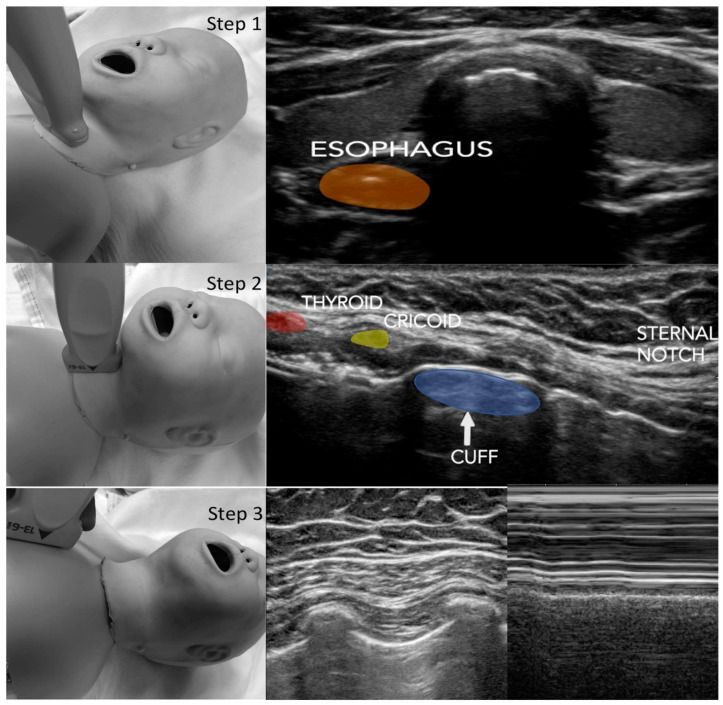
PLUS (pulmonary tree and lung expansion ultrasound study). Step 1: Trachea/Esophagus Assessment—The ultrasound probe is placed transversely on the anterior neck approximately 2 cm superior to the suprasternal notch and scanned cranially/caudad. Step 2: The probe is then rotated 90 degrees such that the long axis of the trachea is achieved with the cricoid cartilage. Step 3: Pleural Sliding Assessment—The ultrasound probe is placed vertically on the anterior chest at the third rib space midclavicular line bilaterally. The assessment of lung expansion is evaluated by the detection of the horizontal movement of the two pleural linings with respiration (lung sliding). The use of the M-mode facilitates the pleural sliding assessment.
